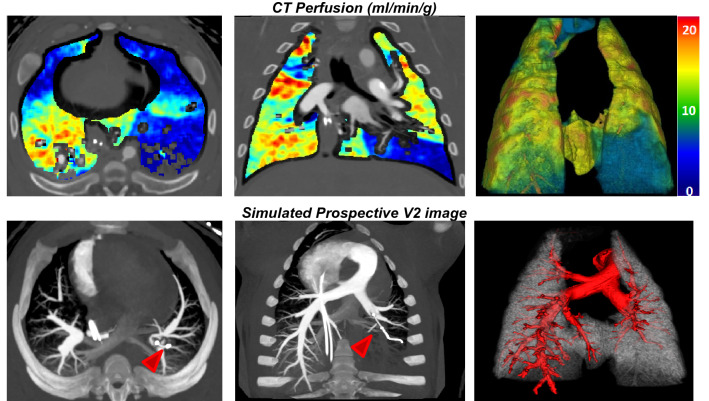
Representative CT perfusion maps and the prospectively predicted V2 image. Top row: Axial, coronal, and 3D posterior views in the presence of balloon occlusions are shown. The color bar on the right indicates the perfusion in the range from 0 to 20 ml/min/g. Bottom row: Axial, oblique-coronal CT angiography using maximum intensity projection reconstruction with 20 mm thickness and 3D pulmonary arteries extraction (Using V2). Red arrows point at the angioplasty balloon.
